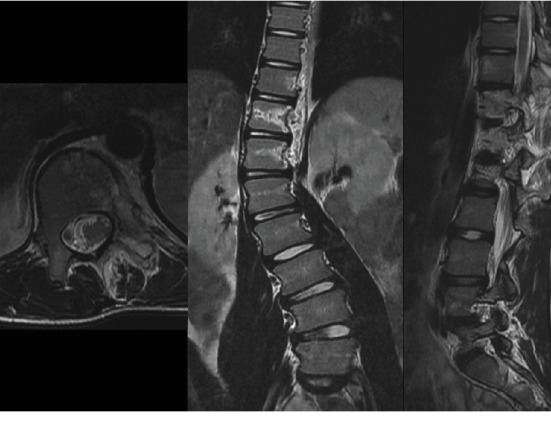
MRI scan at the time of surgical intervention decision. (a) Axial view of T11 vertebral body level. Left side of vertebral body, pedicle, and paravertebral musculature are involved with the tumor. (b) Coronal view. The tumor is located on the left sides of T10–T12 vertebrae, while T11 vertebral body is the most affected by the tumor. (c) Sagittal view. The tumor is dispersed among T11 and T12 vertebral bodies, passing the pedicles, extending to the laminae.
